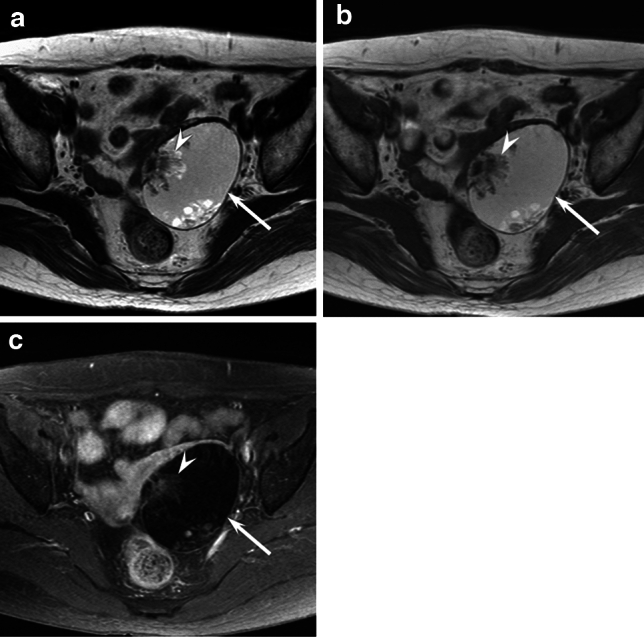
A 66-year-old woman with BMCT. Axial T2-weighted image (a), T1-weighted image (b), and axial fat-suppressed contrast-enhanced T1-weighted image (c) showing a well-defined fat-containing mass (arrow) with endophytic papillary solid components (arrowhead). The occupancy rate of fat components accounting for 93%
